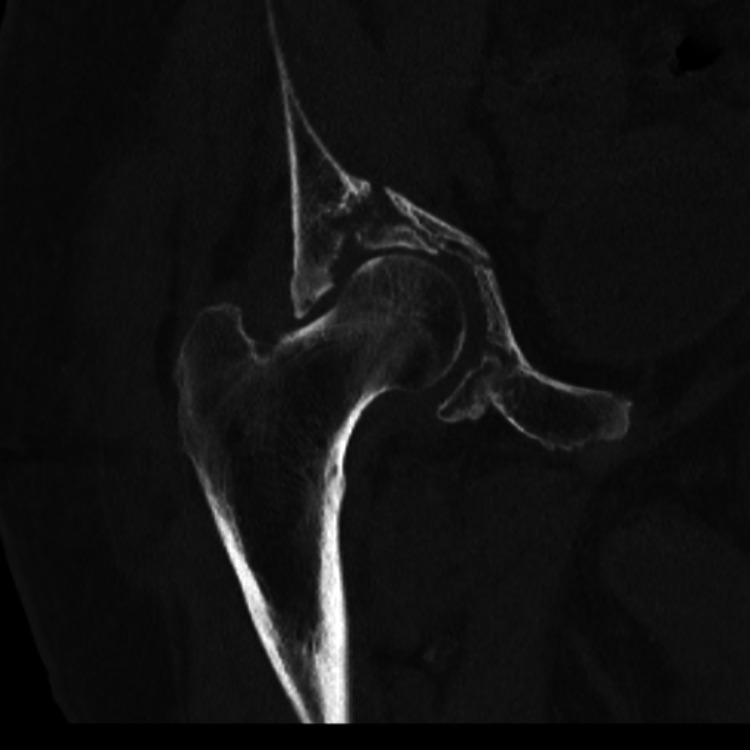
CT scan demonstrating Right Acetabular Fracture Non-Union 5 months post-injury (coronal view)
